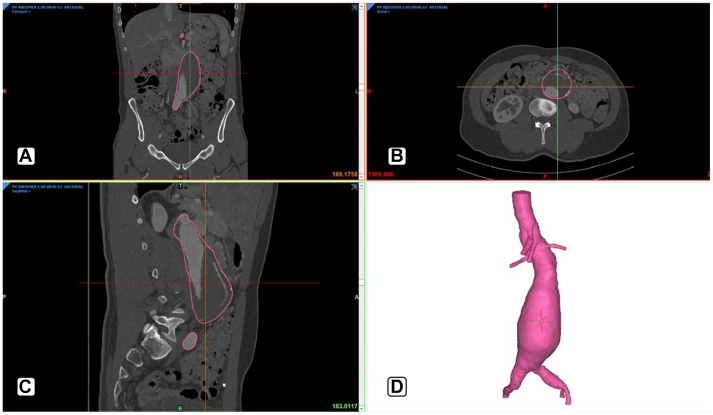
Preoperative computed tomography angiography (CTA) data set of the patient used for model generation: (A) coronal view; (B) axial view; (C) sagittal view; and (D) three-dimensional (3D) segmented reconstruction.
